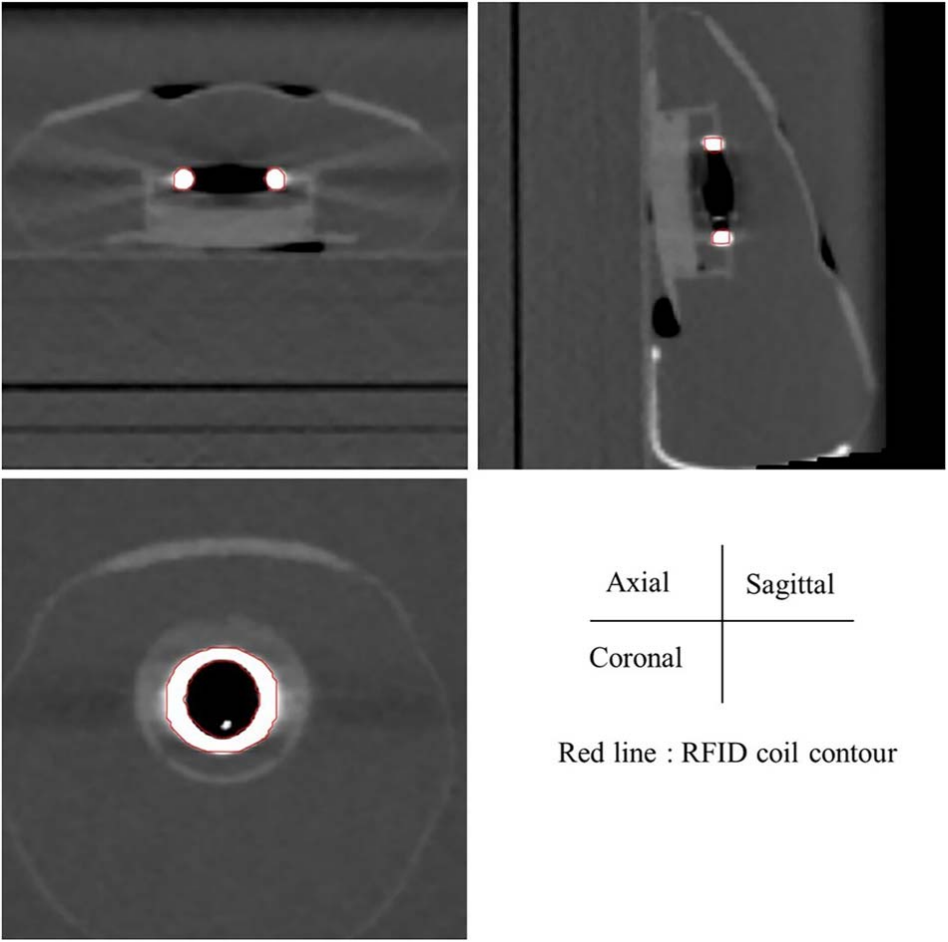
CT image acquired in the modeling study. Lines indicate the coil contour drawn by the ‘Segment High Density Artifacts’ function.
